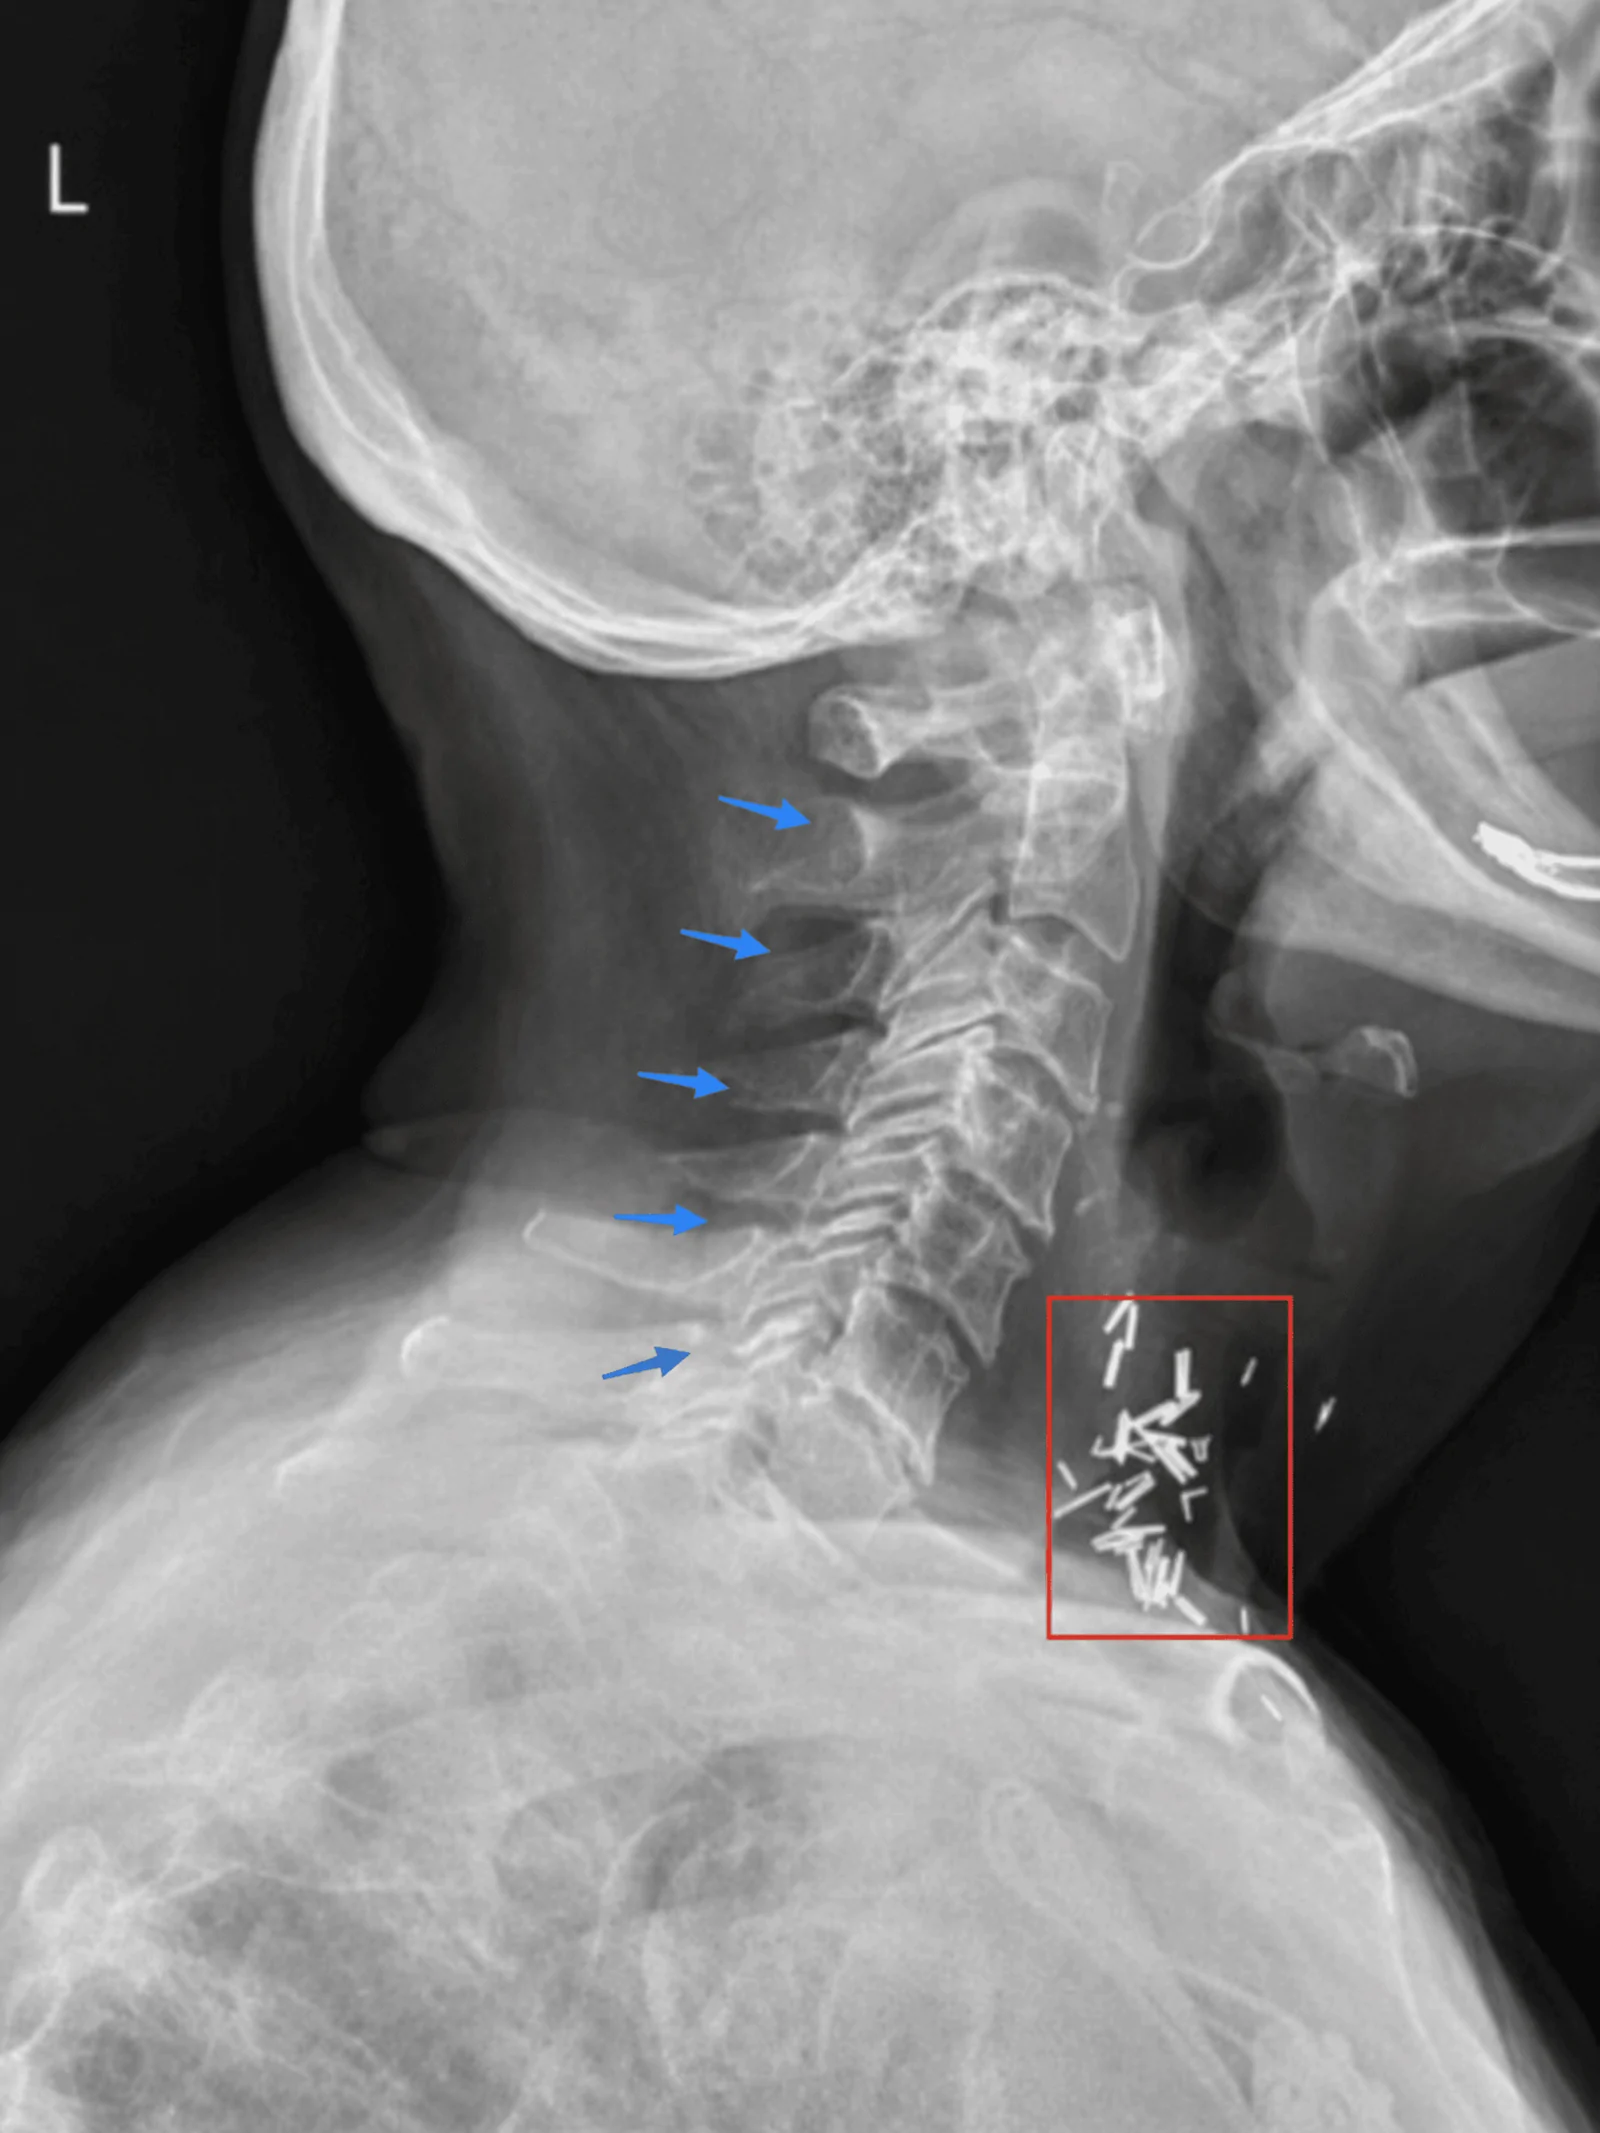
X-ray of the Cervical Spine X-ray of the cervical spine showing no fractures but moderate multilevel degenerative changes (blue arrows). Surgical clips are present in the soft tissue of the neck (red box).
